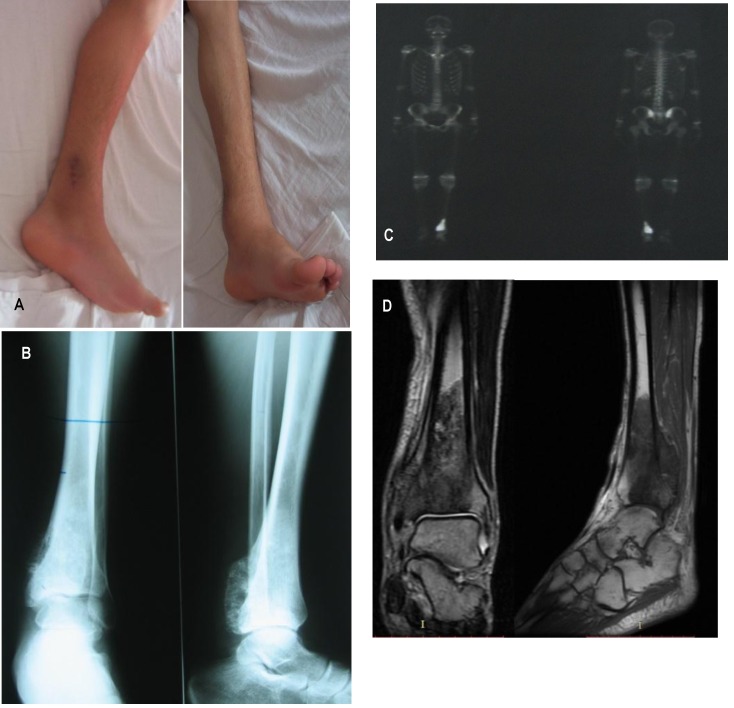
A 17-year-old patient, diagnosed with Ewing sarcoma in the distal third of the left tibia, which invaded the growth cartilage. A. Clinical aspects. B. Preoperative radiological examination. C. Whole body scintigraphy scan - replacement process space well vascularized to the left tibia D. MRI – fusiform heterogeneous tumor mass confined to tibia
